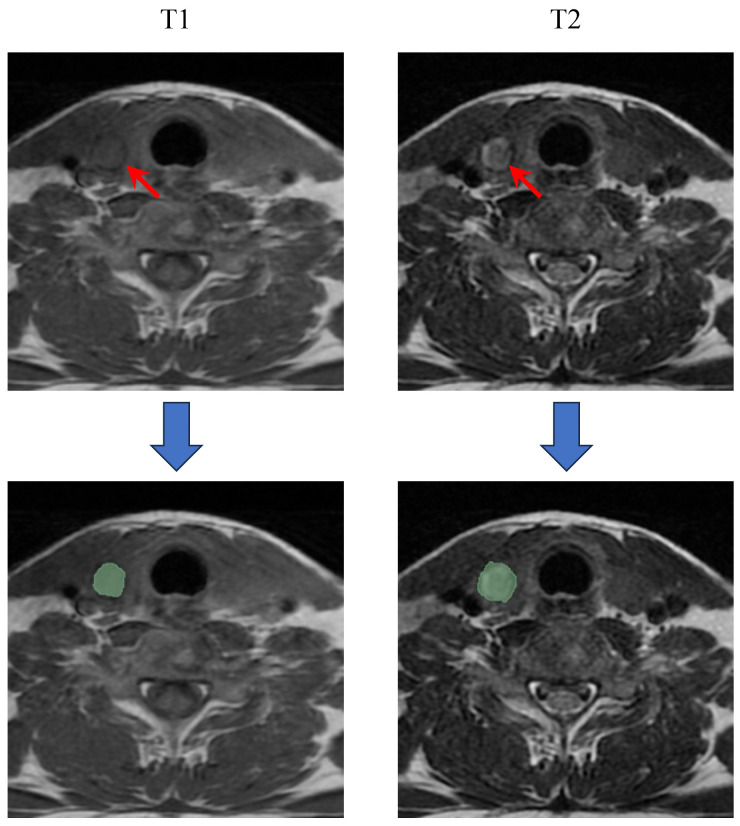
Segmentation of the ROI in axial T1 and T2 images. The red arrows in the MRI images indicate the location of the primary lesion.
